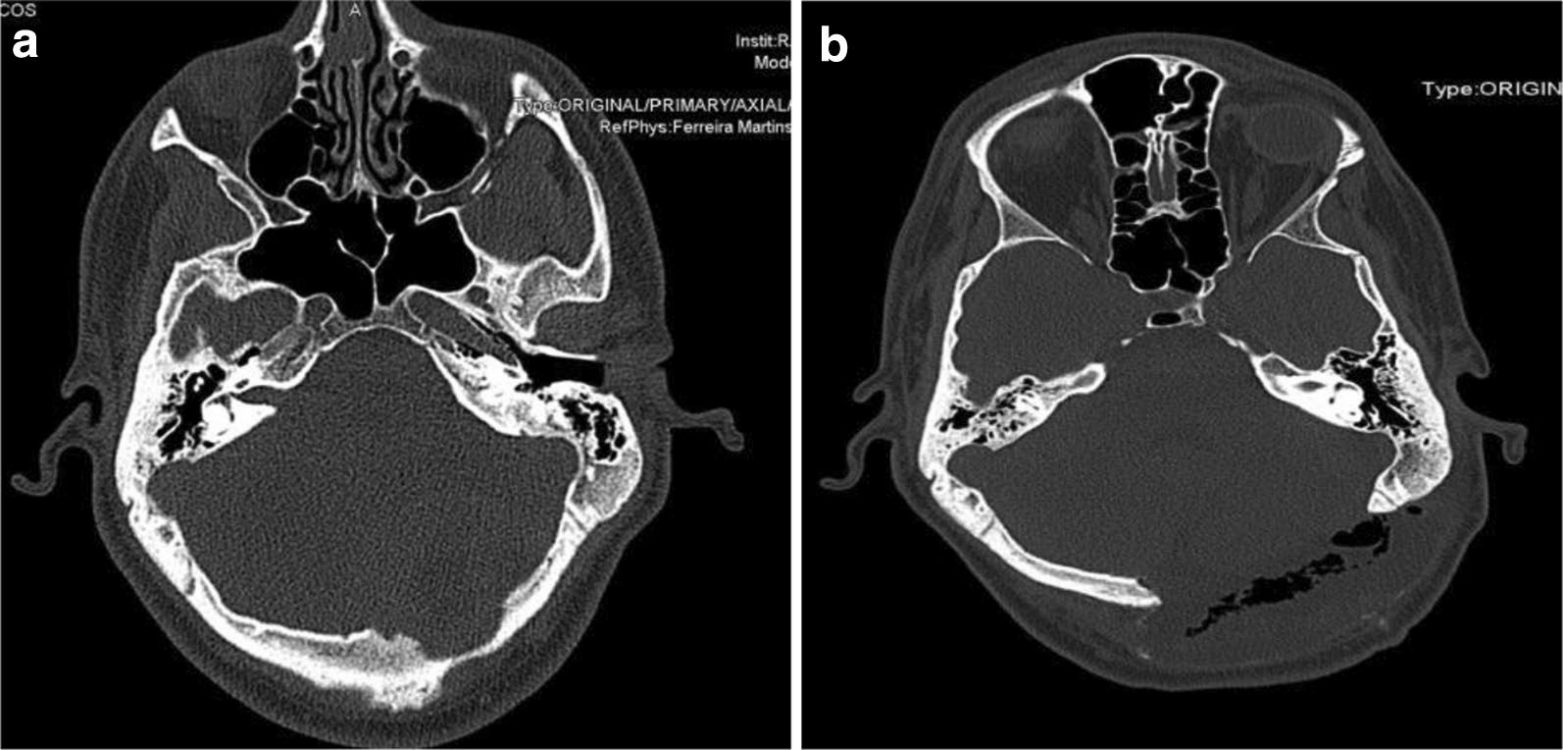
a Preoperative CT scan showing an osteolityc lesion in the occipital bone. b Postoperative CT scan showing bone resection
